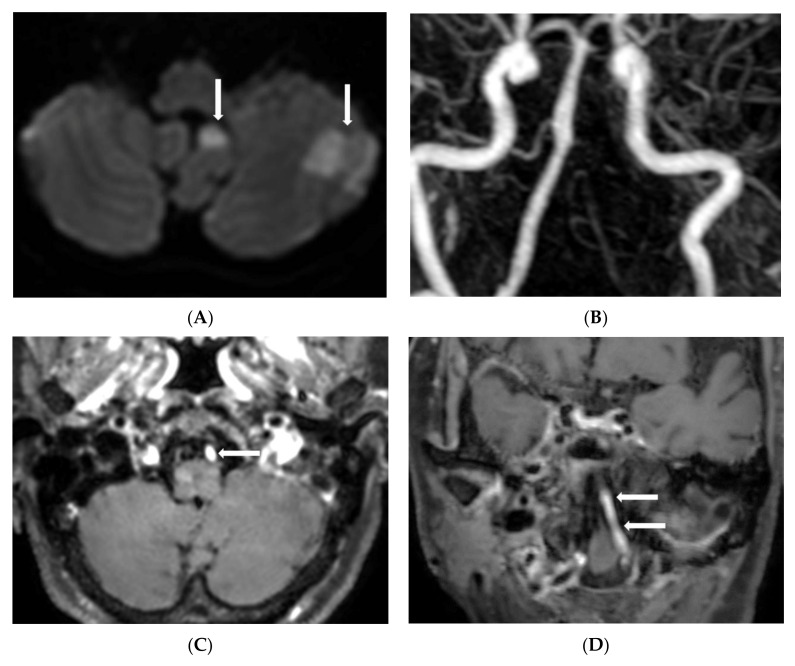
An 83-year-old woman with acute vertigo attack. (A) Diffusion-weighted imaging shows the acute infarction in the left posterior inferior cerebellar artery territory (arrows); (B) Contrast-enhanced MRA shows no visualization of the left vertebral artery; (C) Axial imaging of black-blood enhanced MR imaging shows strong enhancement of left vertebral artery (arrow); (D) Coronal reformatted imaging of black-blood-enhanced MR imaging shows long segment contrast enhancement of left vertebral artery (arrows). This finding suggests contrast stagnation by acute occlusion of the distal vertebral artery related to acute infarction of the posterior inferior cerebellar artery territory.
